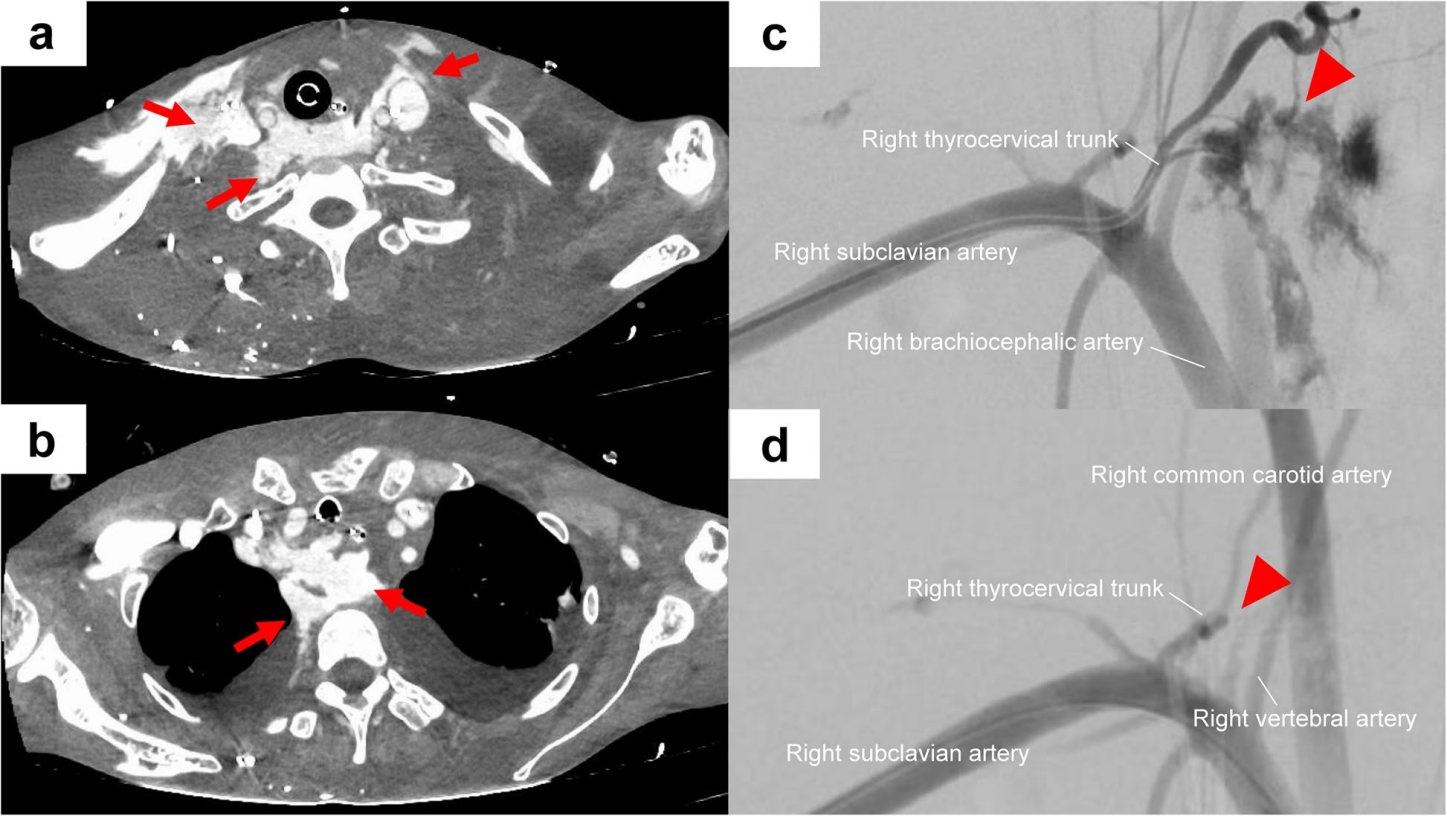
Massive bleeding from a ruptured right thyrocervical trunk associated with right internal jugular vein puncture. Contrast-enhanced computed tomography shows massive contrast media extravasation around the neck (a, arrows) and mediastinum (b, arrows). Successive brachiocephalic artery angiography shows a characteristic “blush” appearance of a ruptured right thyrocervical trunk (c, arrowhead). After selective arterial embolization using 33% N-butyl-2-cyanoacrylate, the extravasation has completely disappeared (d, arrowhead)
